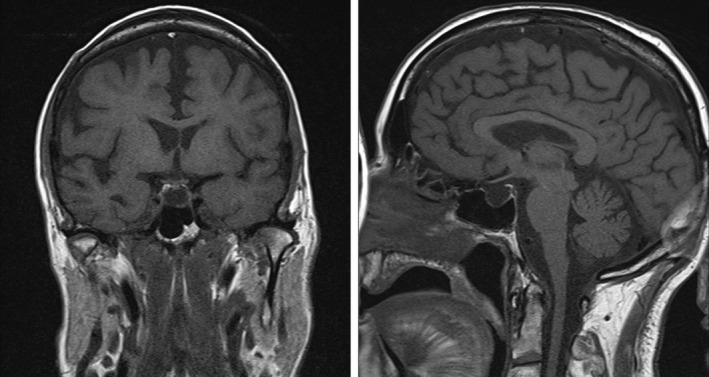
Coronal and sagittal sections of the patient's pituitary magnetic resonance imaging (MRI)
